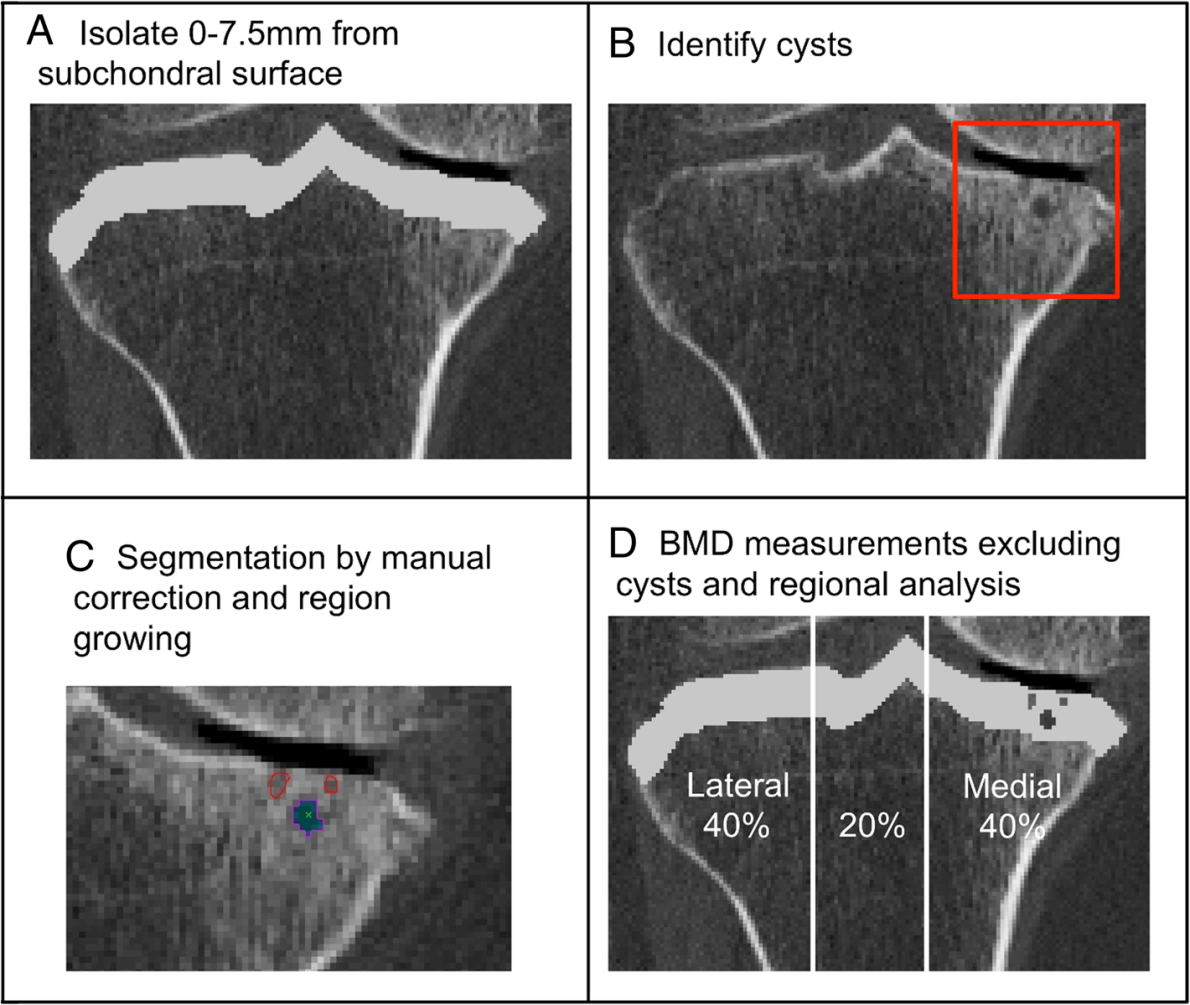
Process for identifying, isolating, and measuring cysts included isolating the subchondral region of 7.5 mm from the subchondral surface (a), cyst identification on CT images (b), and segmenting individual cysts using semi-automatic region growing for larger cysts manual segmentation for smaller cysts (c). Segmented image volumes were then used to measure cyst parameters, and regional BMD excluding cysts which included total, lateral, and medial regions (d)
